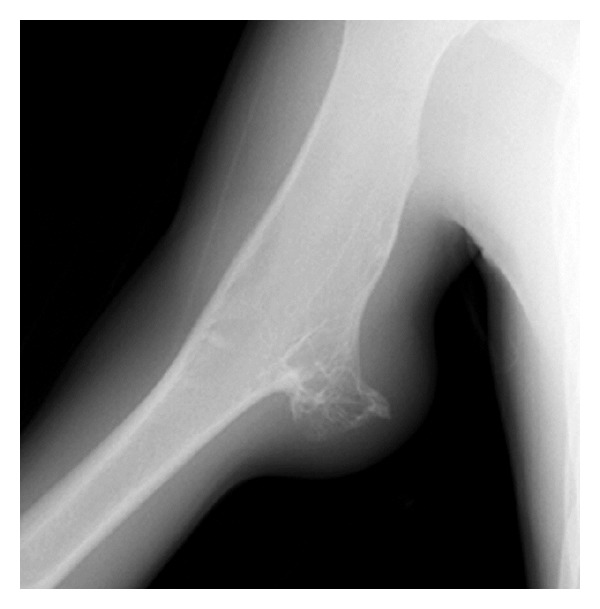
Oblique radiograph of the right arm shows soft tissue swelling overlying a broad-based osteochondroma arising from the humerus and directed towards the chest wall.
